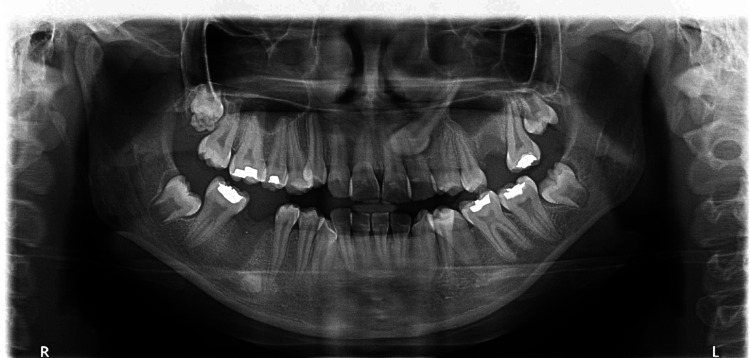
Pre-treatment orthopantomagram radiograph
